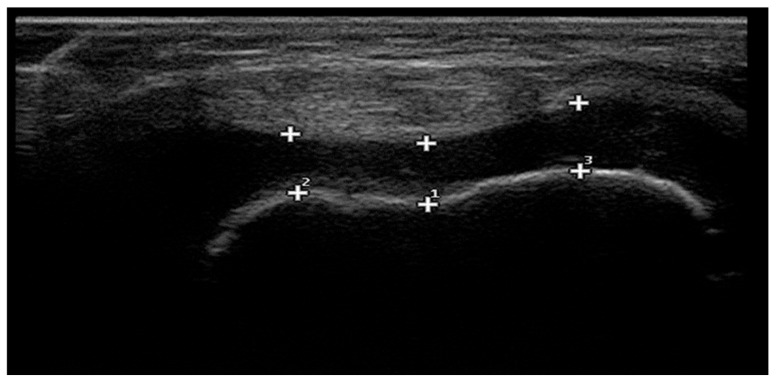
Ultrasound measurement of cartilage thickness. 1: Intercondylar area (ICA), 2: medial femoral condyle (MFC), and 3: lateral femoral condyle (LFC).
